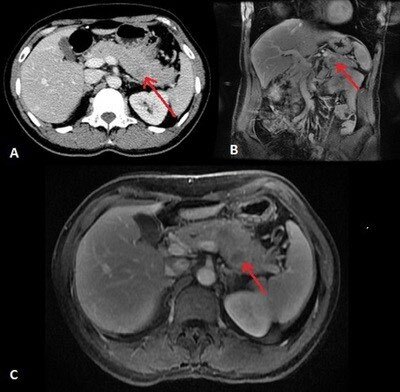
(A) Axial abdominal CT scan showing a hypodense nodule in pancreatic tail; (B) Coronal MRI showing a nodule in pancreatic tail; (C) Axial MRI showing a nodule in pancreatic tail.
